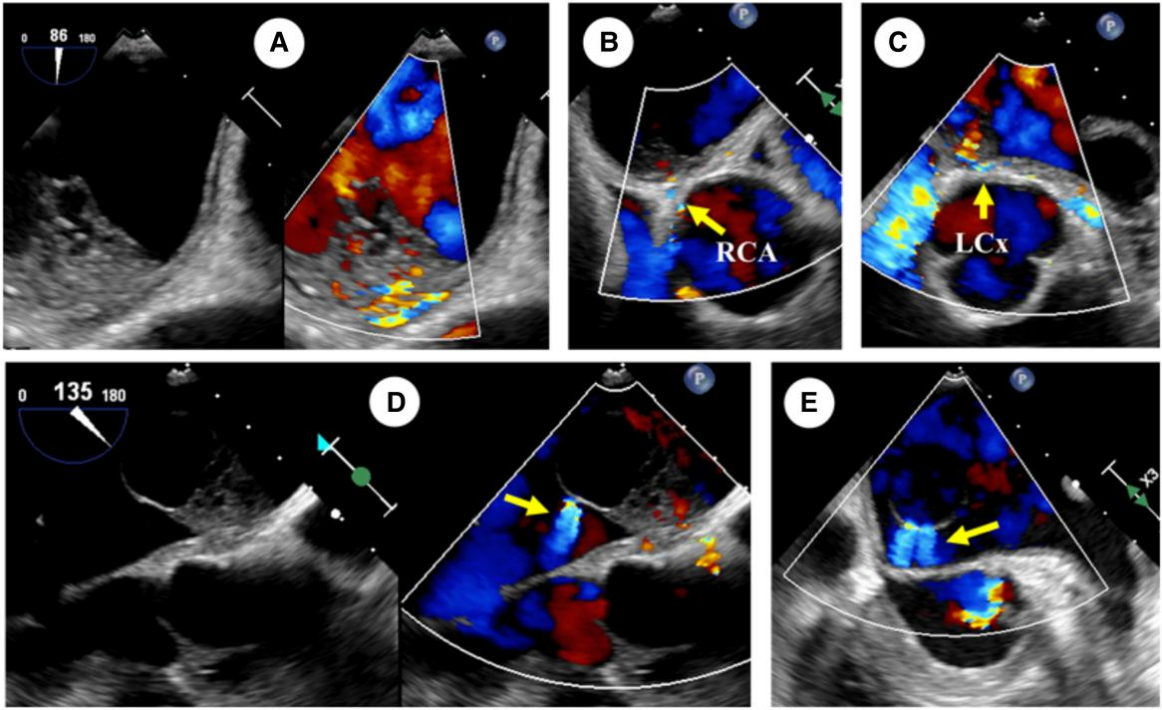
The images of the blood inflow and outflow of the cystic lesion by transoesophageal echocardiography. The Colour Doppler image showed that the feeding arteries flowed from the bottom of the tumour into the cystic lesion (*A*–*C*), and twin jets drained from the cystic part (*D*, *E*). RCA, right coronary artery; LCX, left circumflex branch.
